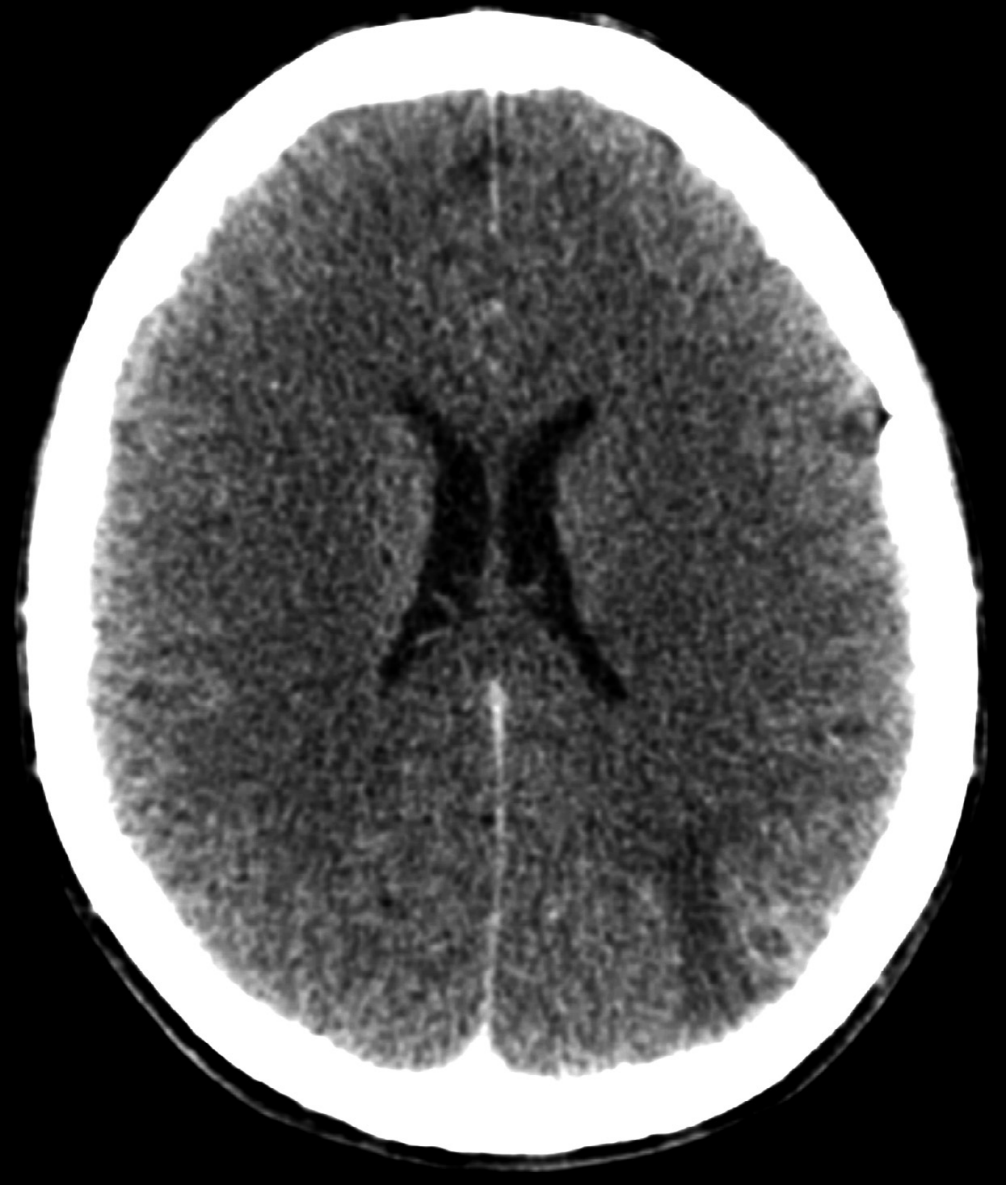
Contrast CT demonstrating hypodensity in Left frontal parietal lobe and Left parietal ring‐enhancing lesion.
